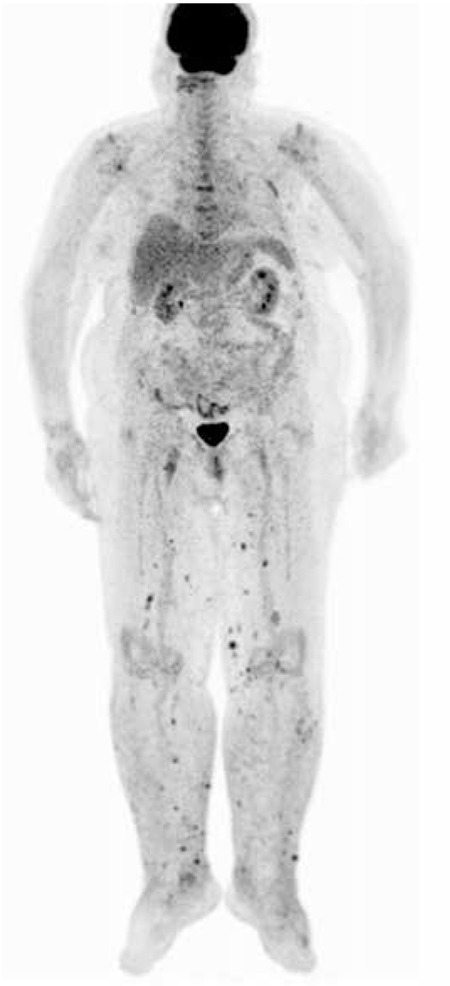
Maximum intensity projection images show multiple nodular lesions with increased fluorodeoxyglucose uptake on the skin and subcutaneous tissues of the legs (SUV_max_: 6.1), and hypermetabolic lymph nodes at bilateral inguinal and popliteal sites
